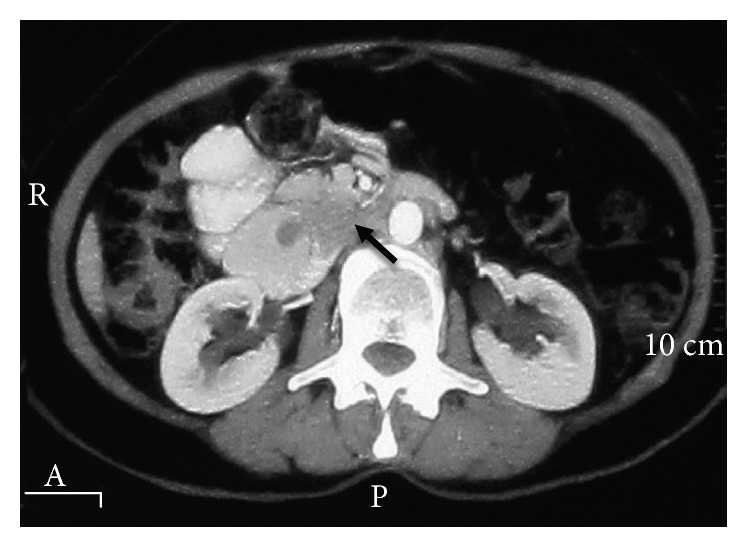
The preoperative abdominal CT scan exhibited a hypodense lesion in the pancreas head and neck (the black arrow indicates the mass).
